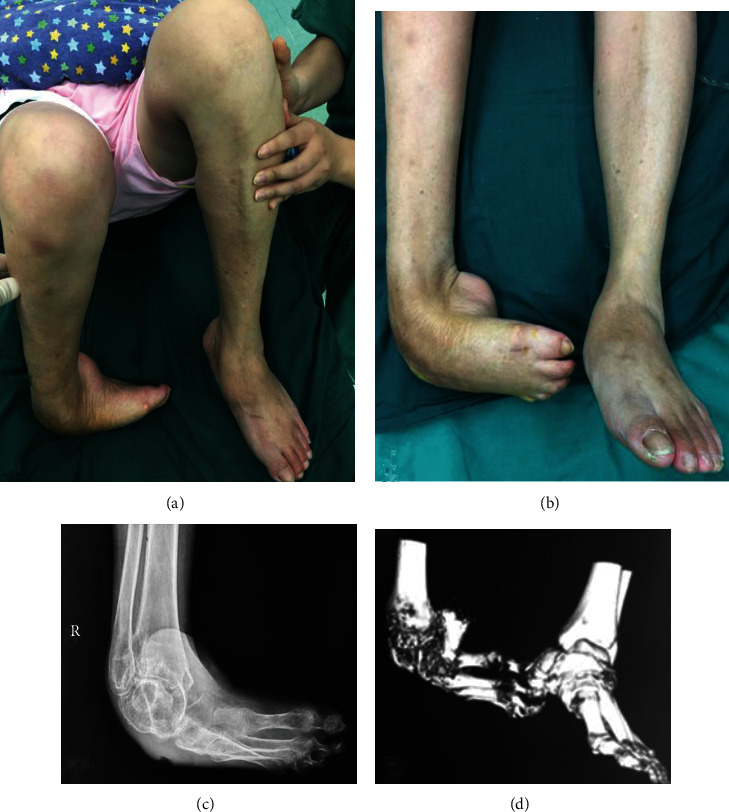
Female, 57 years old, who has suffered from CTE in right side for 50 years. (a) The patient has not paid enough attention to the CTE and walked on the back of foot for 20 years. (b) Preoperative appearance, and the physical examination revealed that the right ankle joint was stiff, the subtalar joint had no range of motion, and the knee tendon reflex was hyperactive. (c) and (d) Preoperative X-ray and 3D reconstruction CT scanning of right ankle joint indicated the severe ATE malformation of the patient.
